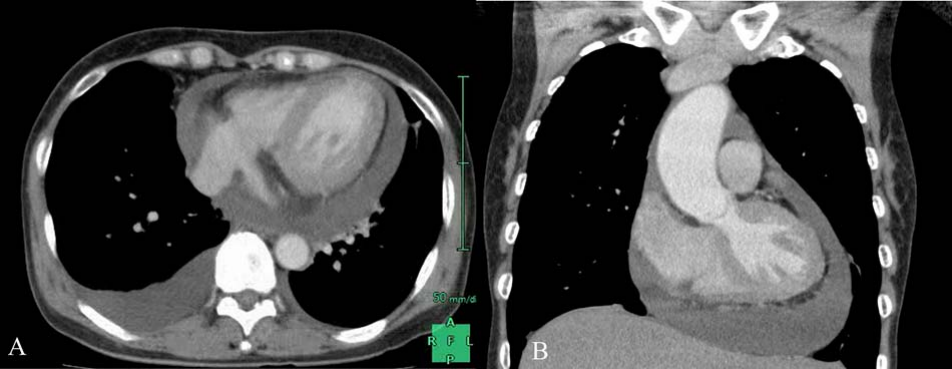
Thoracic and abdominal CT performed at admission; (A) moderate pericardial effusion and right pleural effusion at the transverse section; (B) moderate pericardial effusion at the coronal section.
